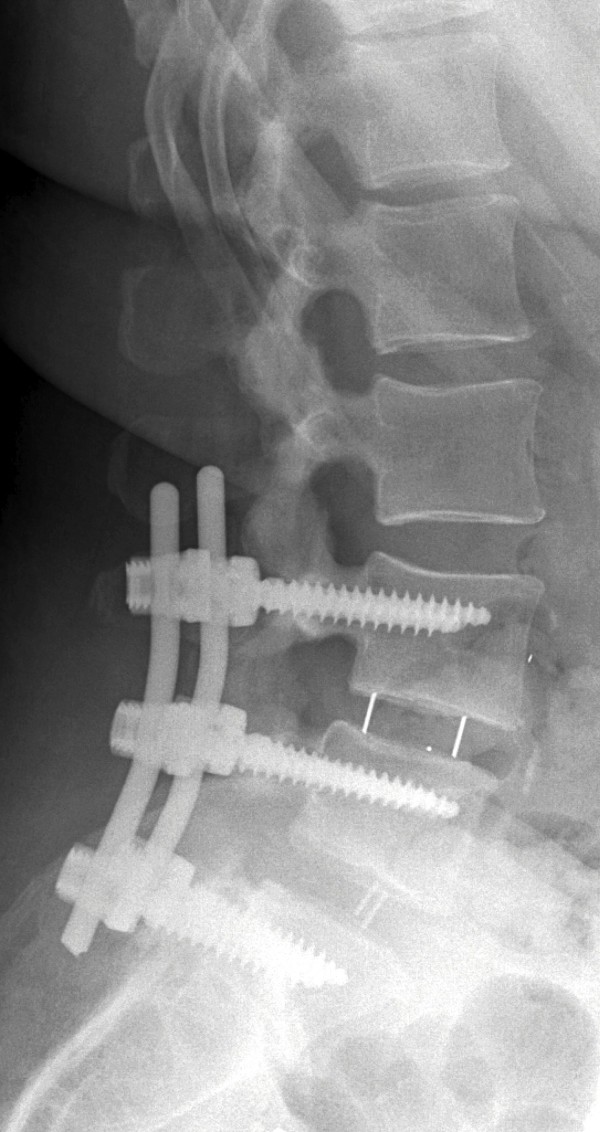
X-ray taken after the patient’s second surgery.
